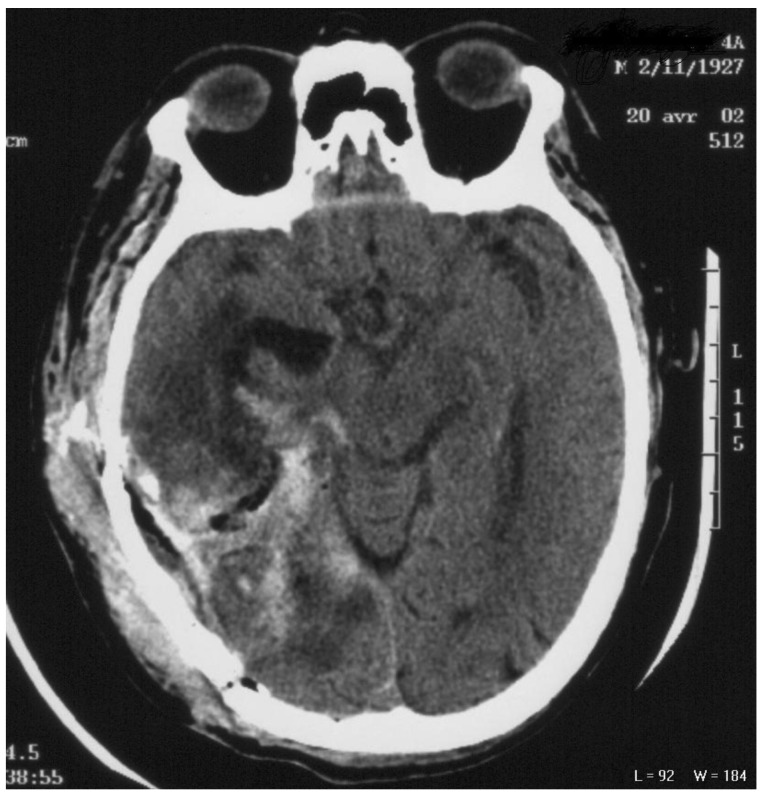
CT-scan axial imaging showing the brain after neurosurgery; a cavity was seen with blood.
